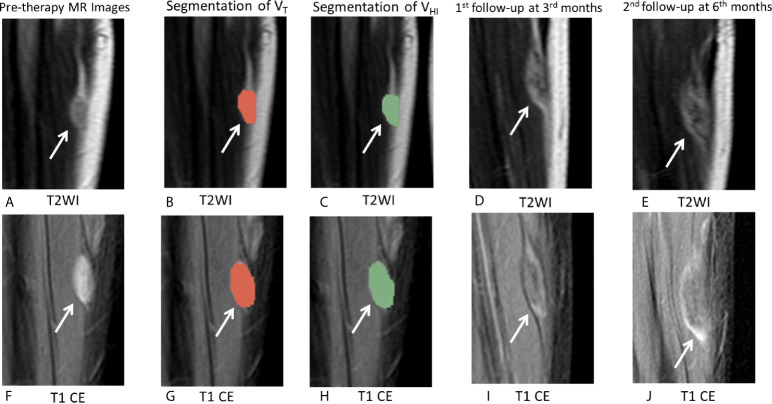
MRI images in a 18-year-old male patient with DF of left calf muscle with imatinib treatment (arrows). Pre-therapy (A, F) diameter, 21.0 mm; On T2WI, V_T_ = 5,477 mm^3^, V_HI_ = 418 mm^3^, HI = 7.5% (grade = 0); On T1 CE, V_T_ = 5,238 mm^3^, V_HI_ = 5,238 mm^3^, HI = 100% (grade = 3). Segmentation of V_T_ (B, G, red zonex) and segmentation of V_HI_ (C, H, green zone). First follow-up at 3 months since treatment with imatinib (D, I) diameter, 25.0 mm indicates a 19.0% increase in tumor size. The tumor response assessment is SD, according to RECIST1.1. Second follow-up at 5 months since treatment with imatinib (E, J) diameter, 36.0 mm indicates a 71.4% increase in tumor size. The tumor response assessment is PD, according to RECIST1.1.
